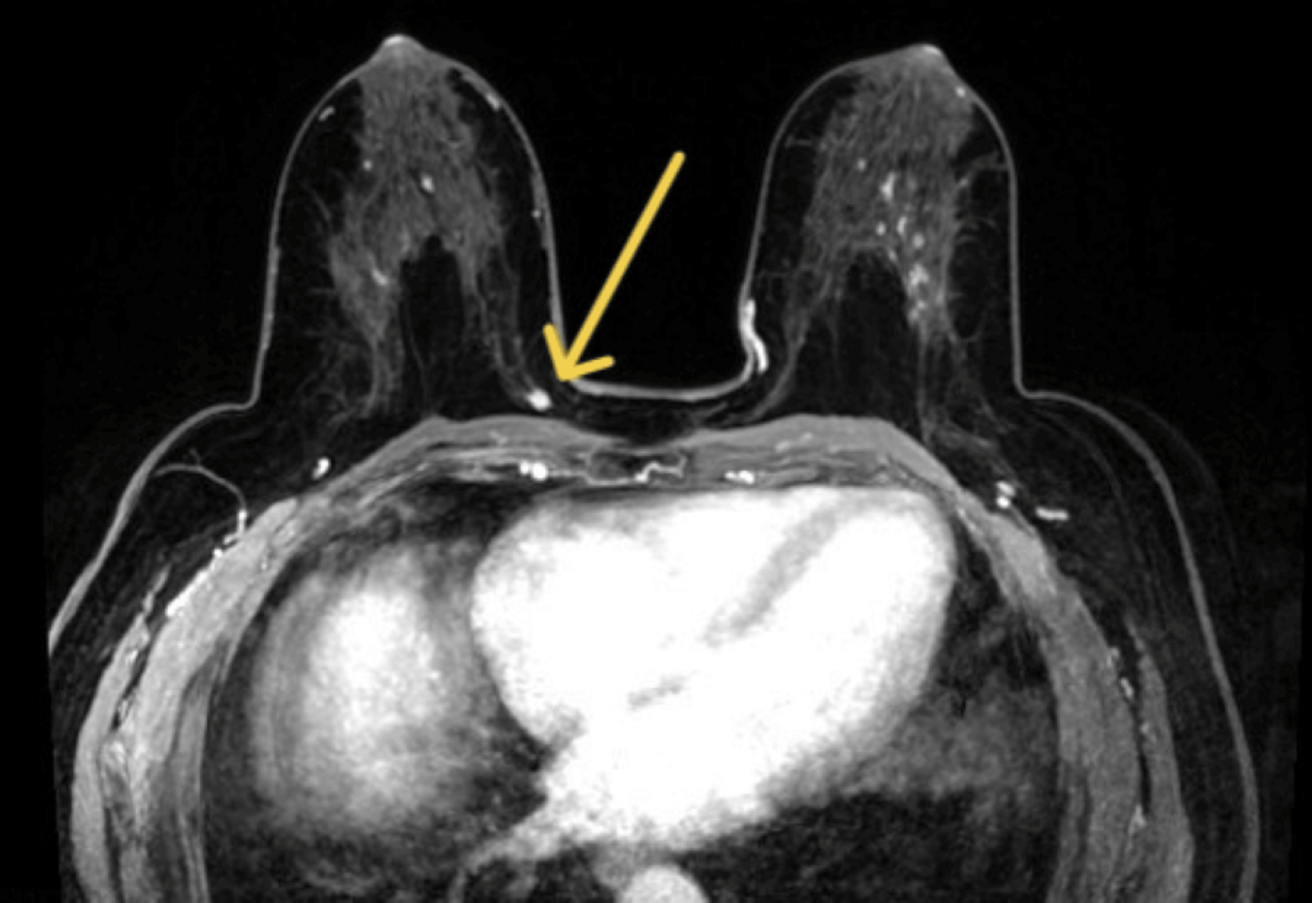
MRI showing the primary tumor (yellow arrow), a 6 mm nucleus between the inner quadrants, in a deep location separated from the thoracic wall by 2 mm, and adjacent to the intermammary groove.
